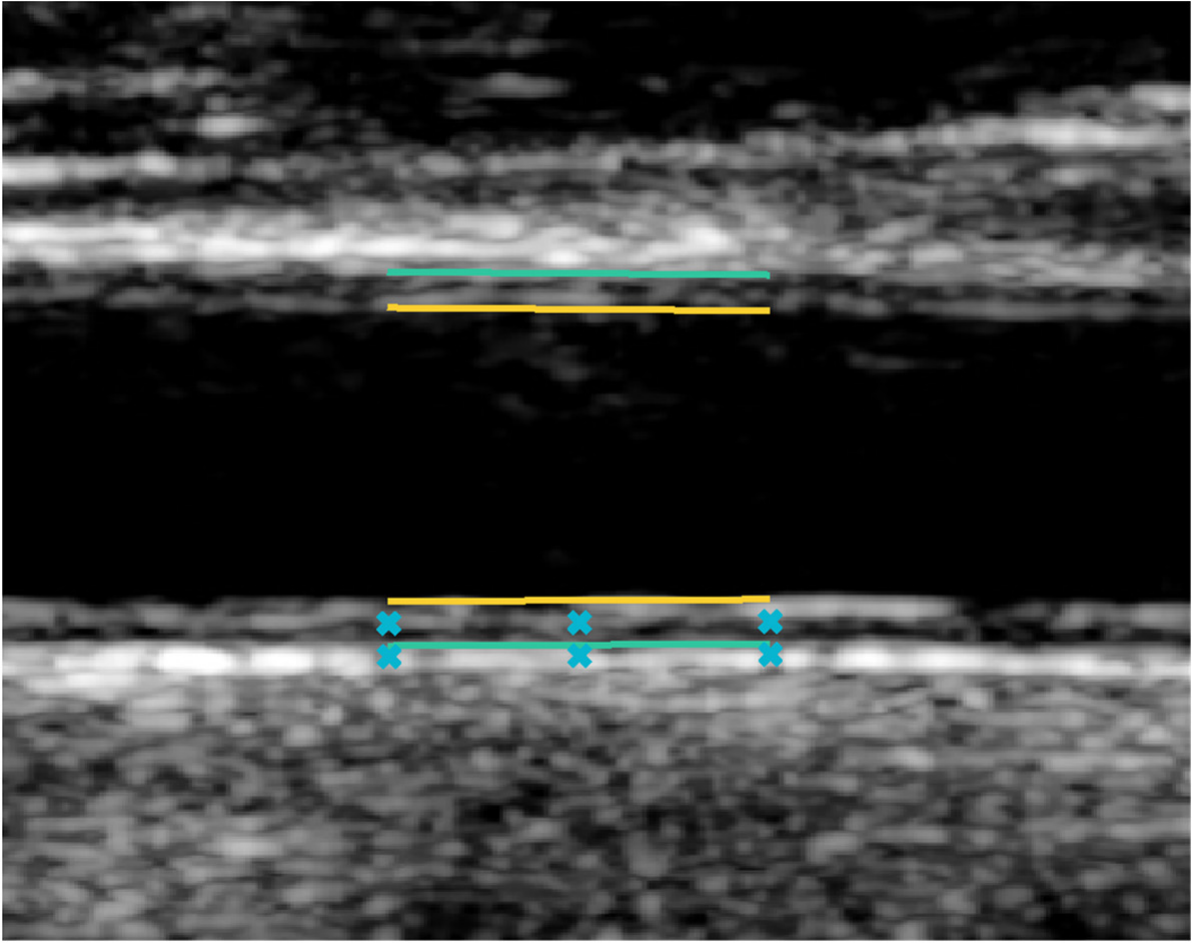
Ultrasound image of the common carotid artery. The yellow lines mark the demarcations between lumen and tunica intima and the green lines the demarcation between media and adventitia. The lines are 5 mm wide. In this study we measure the longitudinal movement at the far wall between the yellow and the green lines, and the shear strain between the blue crosses at intima‐media and adventitial layers.
